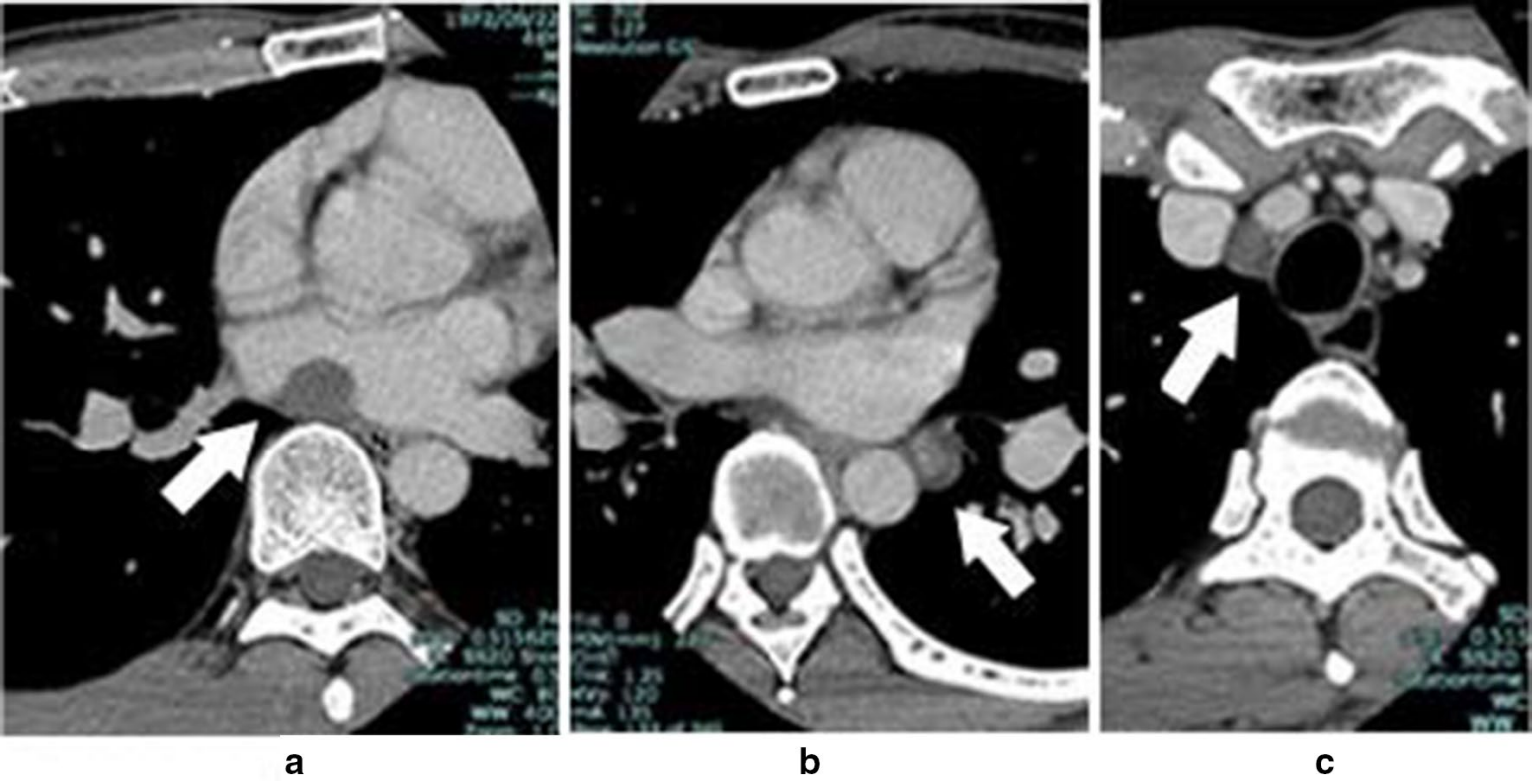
Computed tomography. Computed tomography reveals three masses: in the posterior mediastinum (a), left pulmonary hilar area (b), and right pulmonary apex area (c)
